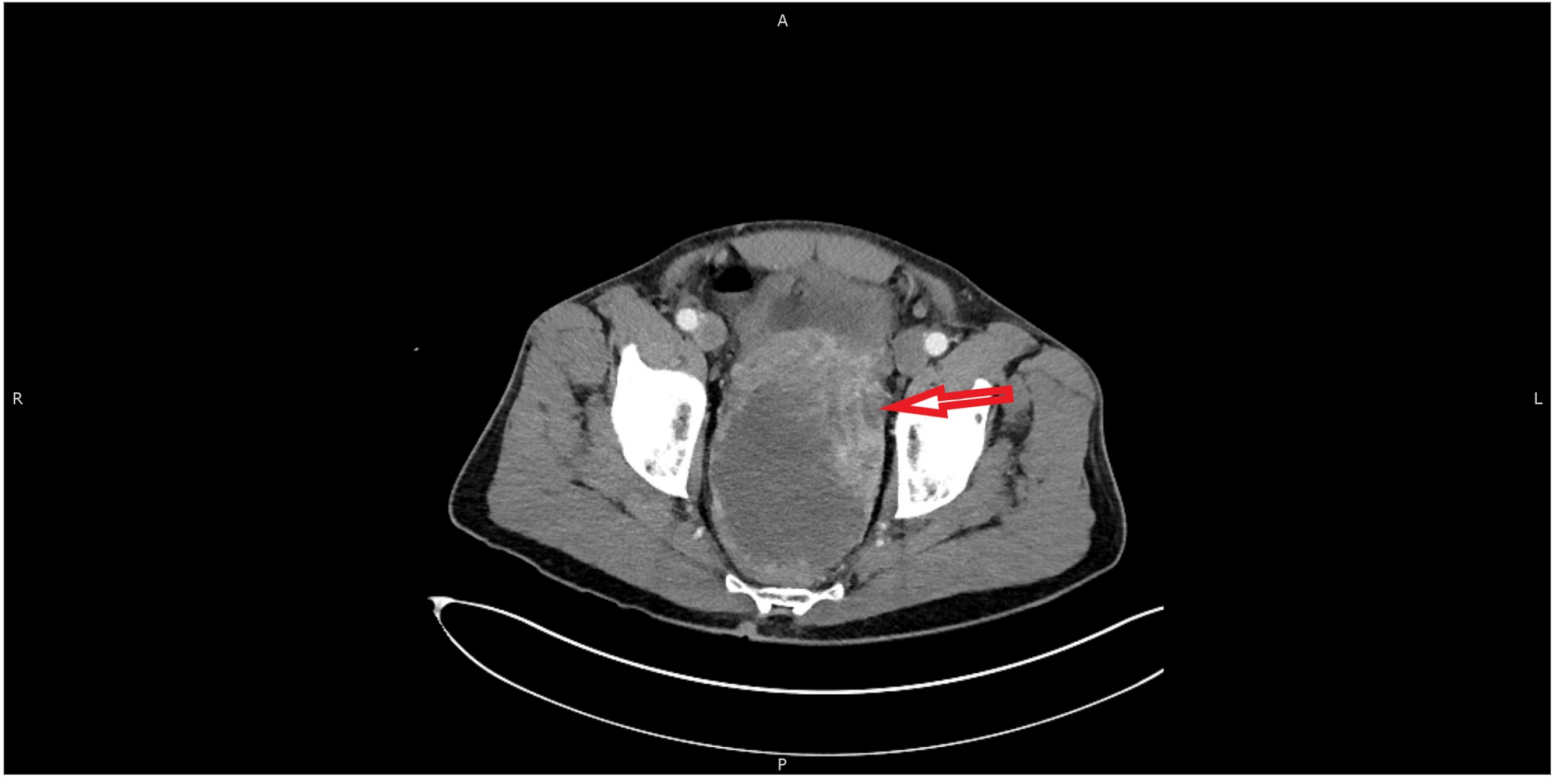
MRI (axial view) of the pelvis with contrast showing large pelvic mass fistulizing with the rectum (red arrow)
